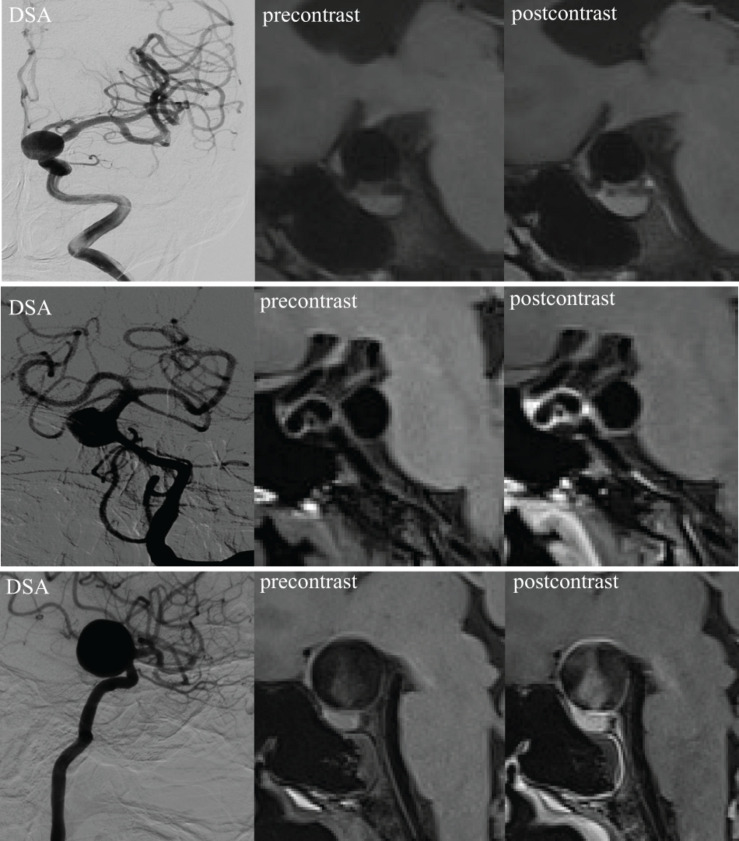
3D high-resolution MRI demonstrating an aneurysm wall without enhancement (pattern 0, first row), focal enhancement (pattern 1, second row), and circumferential enhancement (pattern 2, third row). DSA, digital subtraction angiography.
